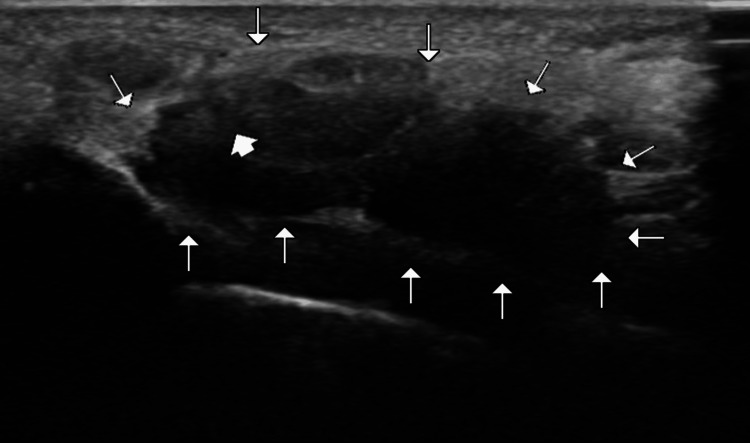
Ultrasound image of the carpal tunnel The carpal tunnel is marked by arrowheads and the median nerve is marked by a thick arrow.
